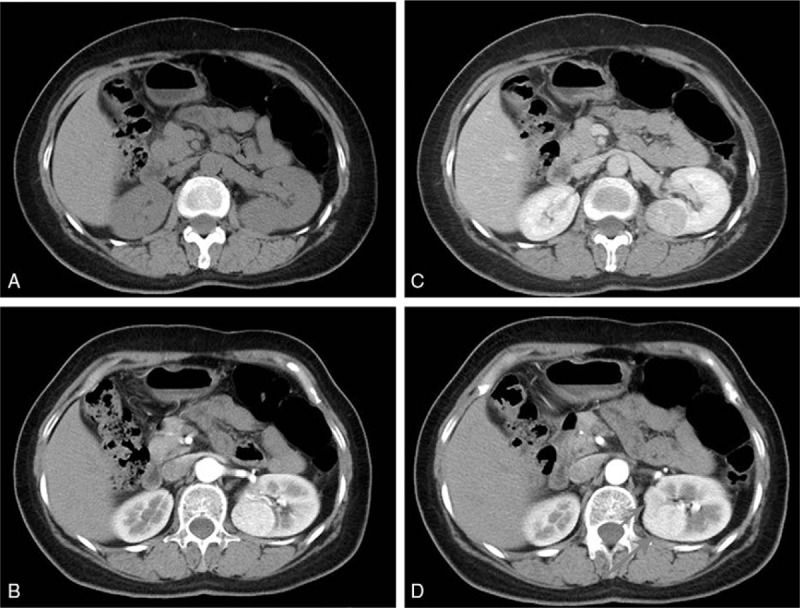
Preoperative CT of our patient. (A) Plain scan shows a large mass measured 3.5 × 3.1 × 3.2 cm in left kidney. (B) The mass has an apparently enhancement in arterial phase. (C) The mass shows a relatively low density compared with parenchyma in venous phase. (D) The multiple renal masses in arterial phase. The masses are pointed out by the red arrows.
